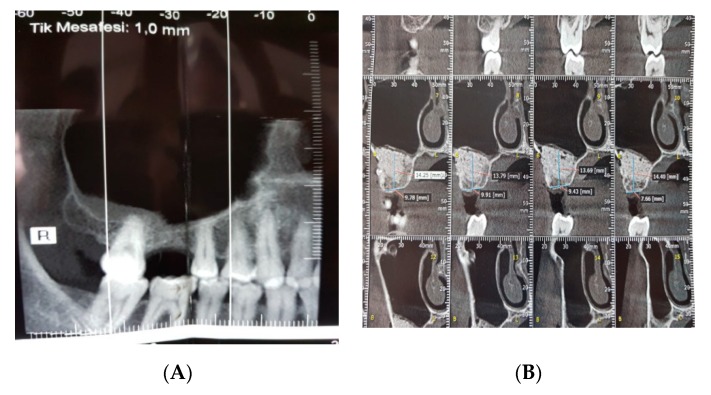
Preoperative (A) and postoperative (B) radiographic view.
